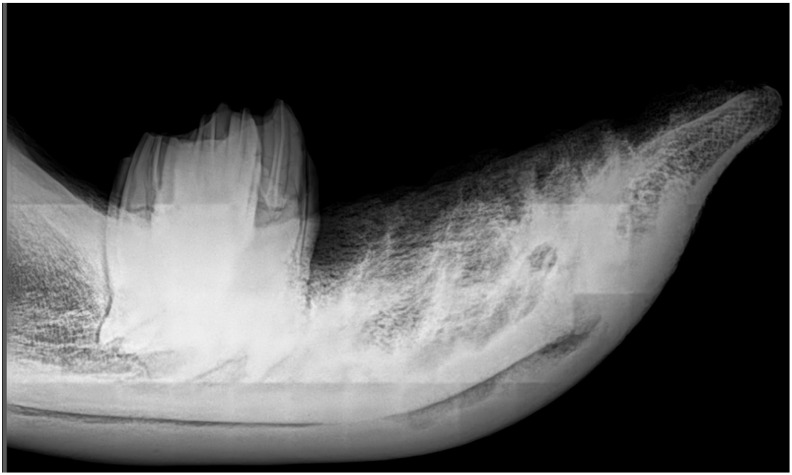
Lateral view X-ray of the mandible of the white rhinoceros female (only M_3_ teeth firmly fixed in alveoli left for imaging).
